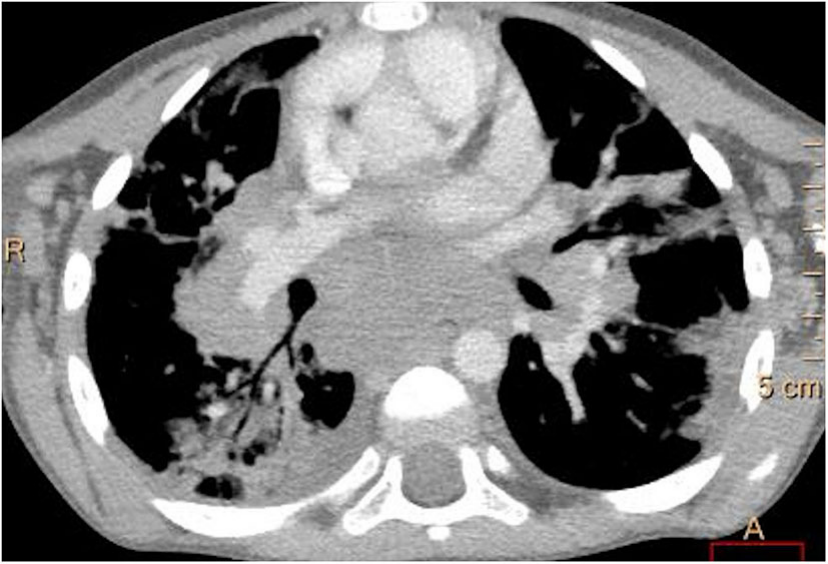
Contrast-enhanced lung computed tomography (soft tissue window): marked enlargement of mediastinal and hilar lymph nodes with areas of subpleural lung consolidations.
